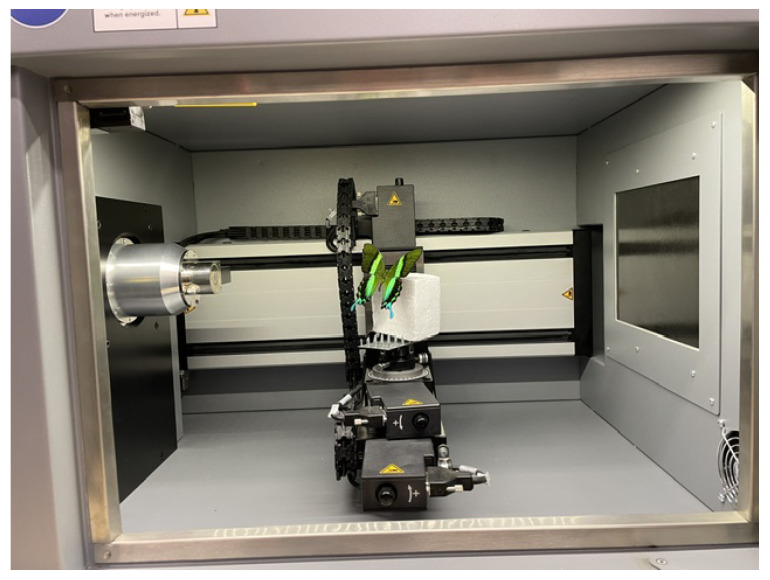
Creating a reference scan (DeskTom CT scanner) [26].
